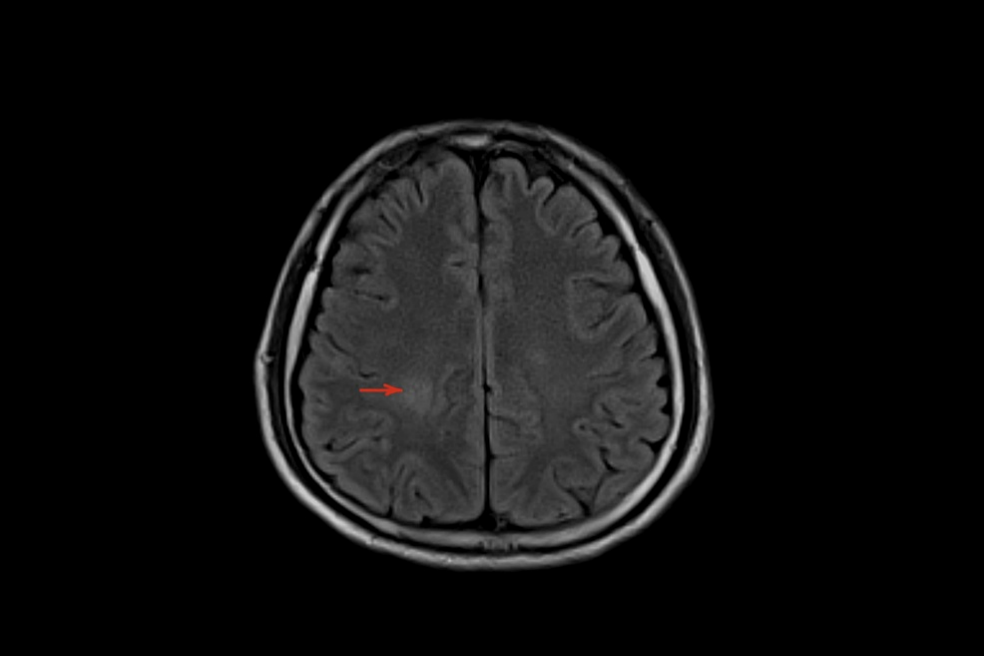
MRI of the brain: FLAIR signal hyperintensity in the right posterior parietal regions in the periventricular deep white matter (red arrow). MRI - magnetic resonance imaging; FLAIR - fluid-attenuated inversion recovery
